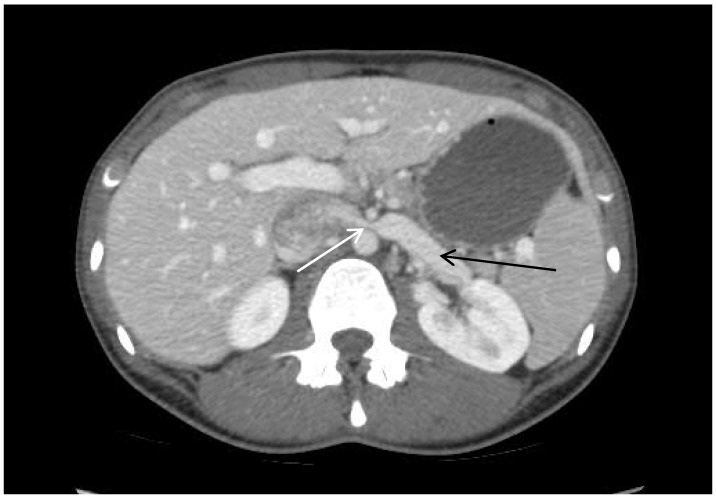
Nutcracker anatomy on computed tomography. White arrow points to compression of the left renal vein (LRV) between the superior mesenteric artery (SMA) and aorta. The black arrow points to LRV dilation.
